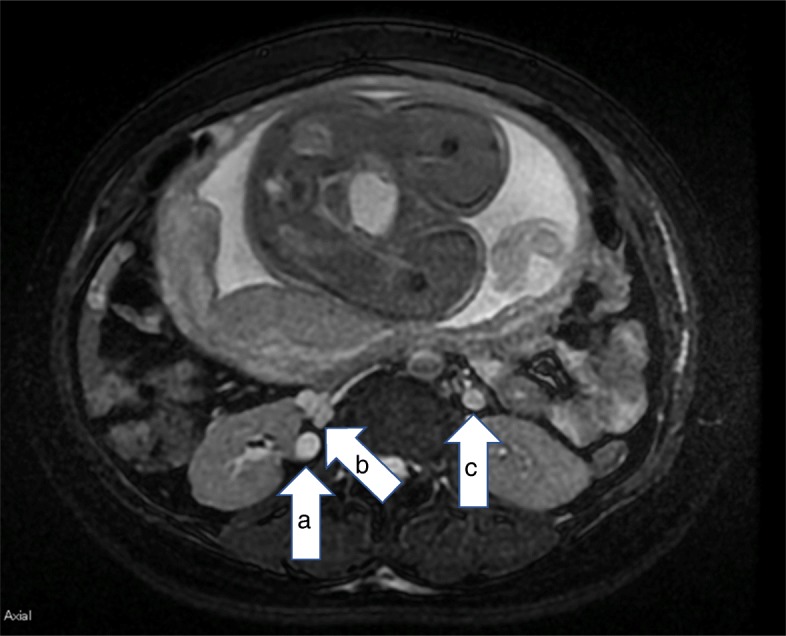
Axial abdominal T2-weighted MRI. a Dilated right ureter. b Inferior vena cava. c Left ureter. Legend: the right ureter (a) traveled to the dorsal side of the inferior vena cava (b) and was compressed by an enlarged fetus, causing hydronephrosis. The dilation of the left ureter (c) was less than that observed in a and is a physiological change seen in pregnant women
